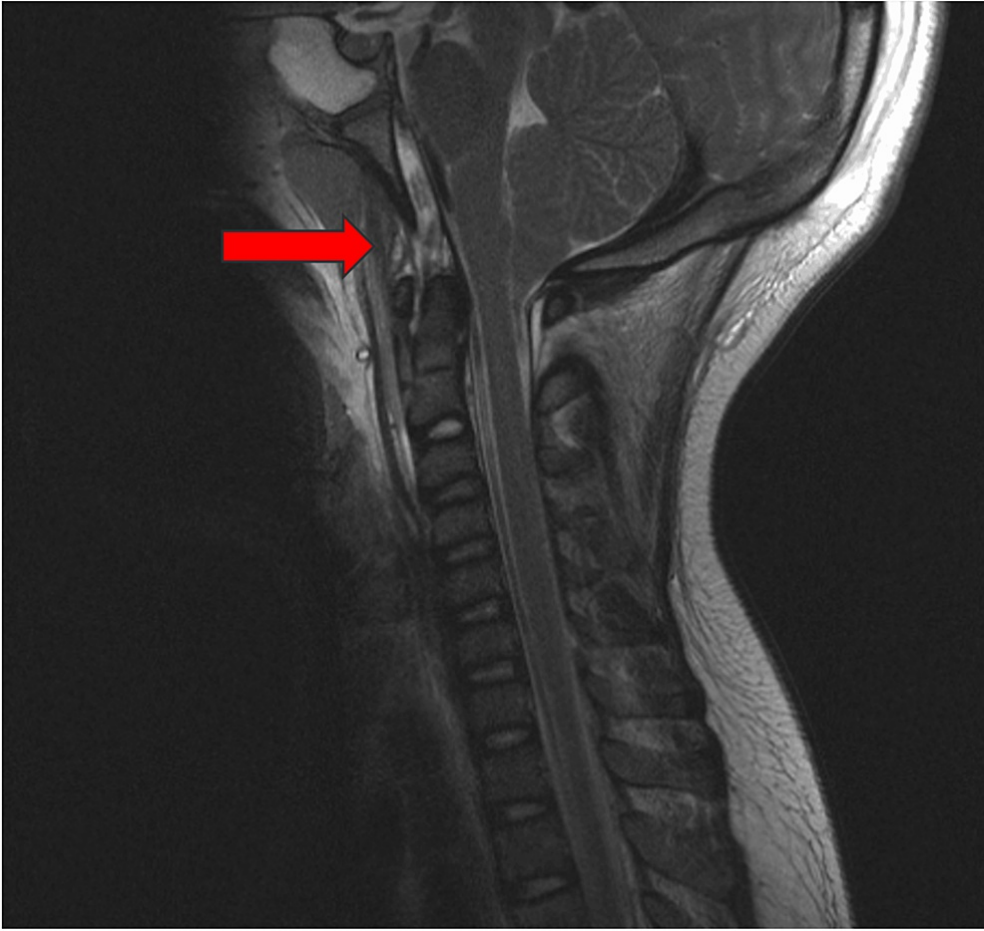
T2 MRI of a 9-year-old patient demonstrating questionable integrity of the ligamentous complex.
